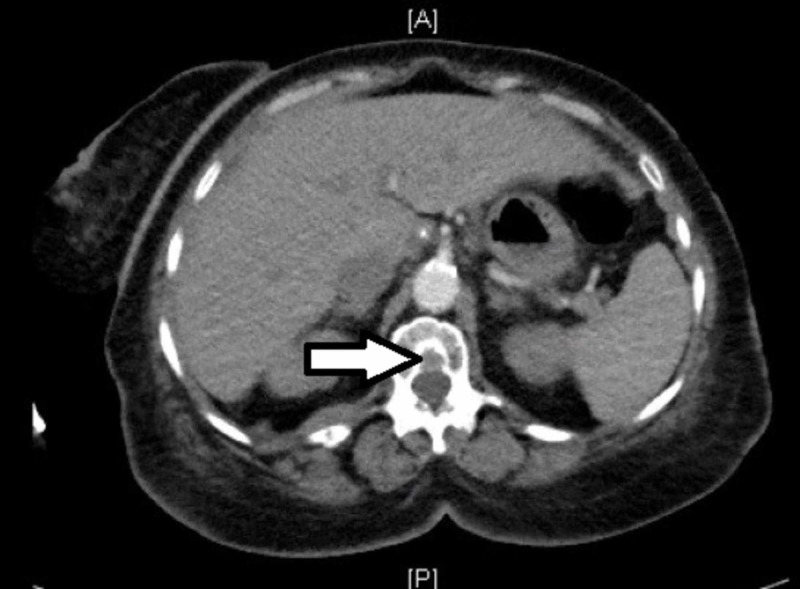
Arrow demonstrating vertebral metastasis on computed tomography scan.
